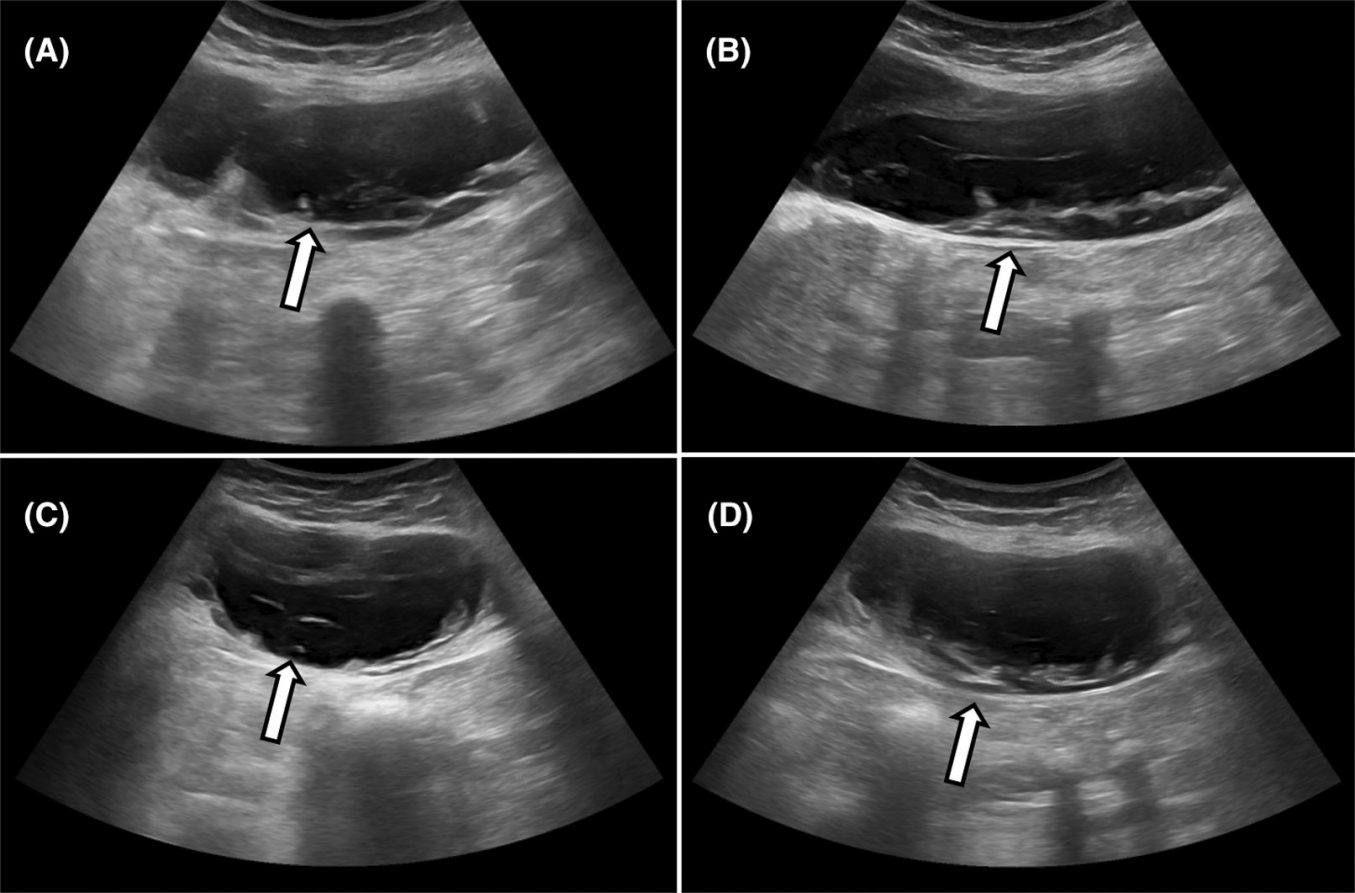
Ultrasonography (A, B, C, D) images showing heterogeneously anechoic lesion (white arrows) replacing right rectus abdominis muscle with few septations and internal echoes.
